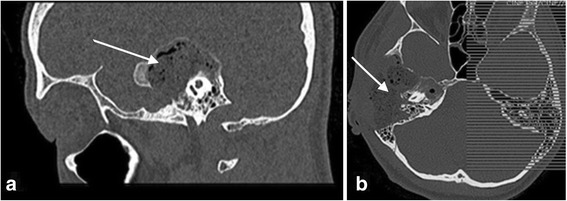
a Postoperative sagittal CT scan shows a large cavity after petrosectomy obliterated with the temporalis muscle. b Axial CT scan shows a large cavity after petrosectomy in the right temporal bone
